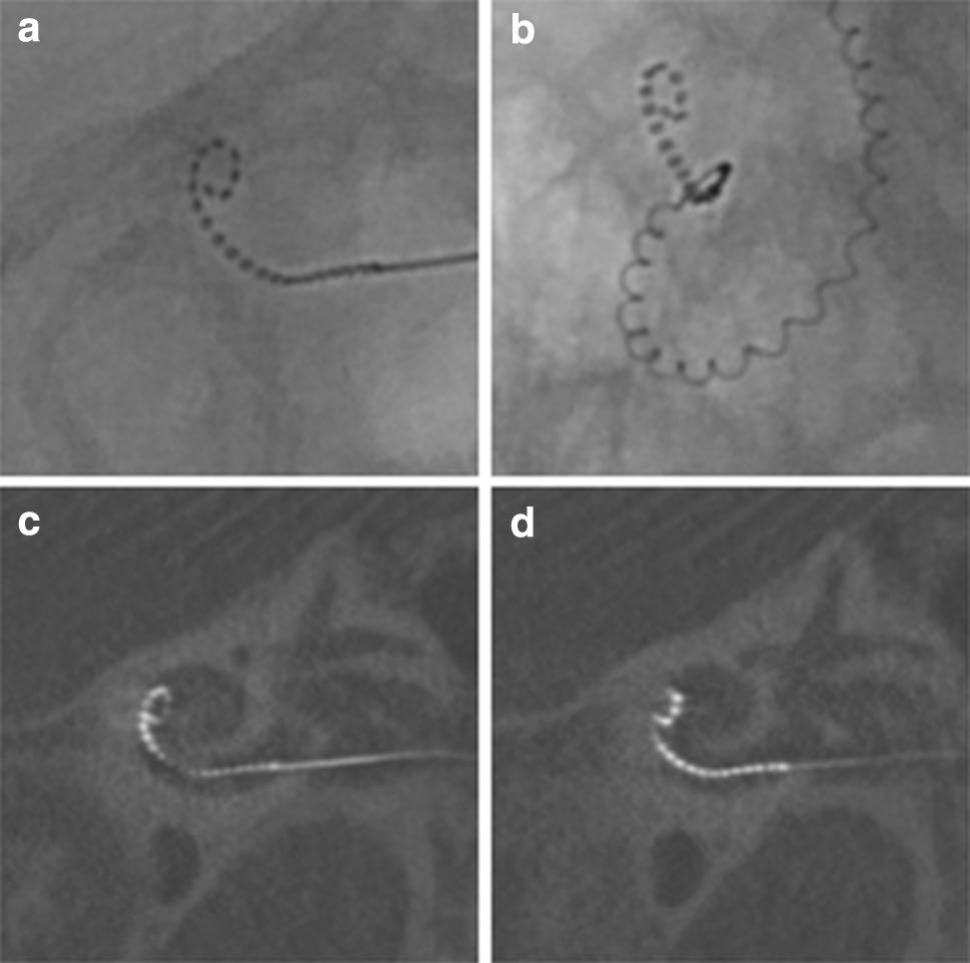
Images of the patient with a tip fold-over (a, b fluoroscopy, c, d CT-scan)
